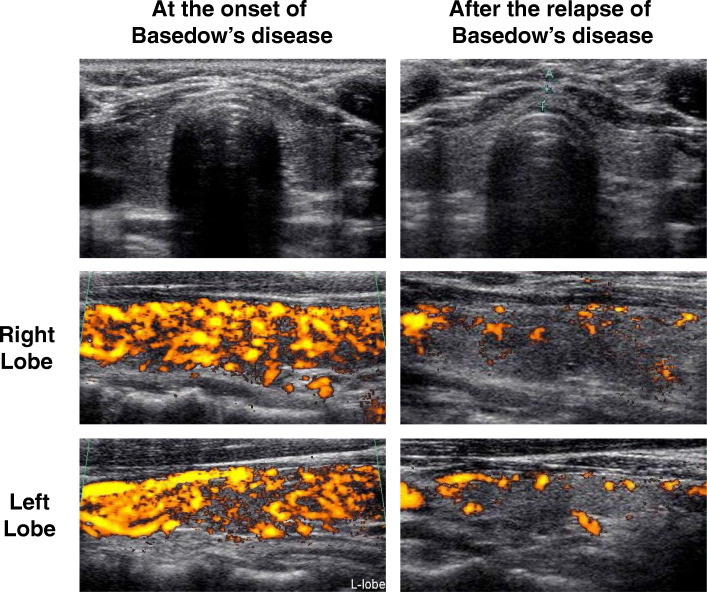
Ultrasound examination at the onset of Basedow’s disease revealed that the thyroid gland was hypervascular although it was not enlarged. Ultrasound examination after the relapse of Basedow’s disease revealed that the thyroid gland was not very hypervascular compared to that at the onset of the disease
